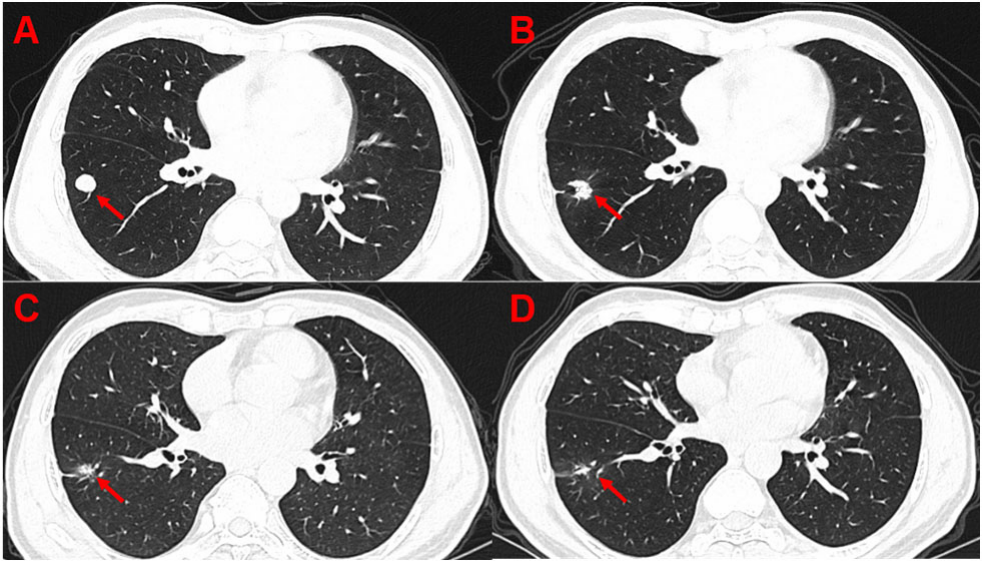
Serial CT images of a representative HCC patient with lung metastases, demonstrating the effect of combined PD-1 inhibitor and ^125^I seed implantation therapy. (A) Baseline scan before treatment shows a lung metastatic lesion (arrowed). (B) 3 months after combination therapy, the lesion has markedly decreased in size. (C) 6 months post-treatment, the lesion is further reduced. (D) 12 months post-treatment, the metastatic tumor has shrunk to near-complete resolution. No new lesions or local recurrence were observed at the 12-month follow-up.
